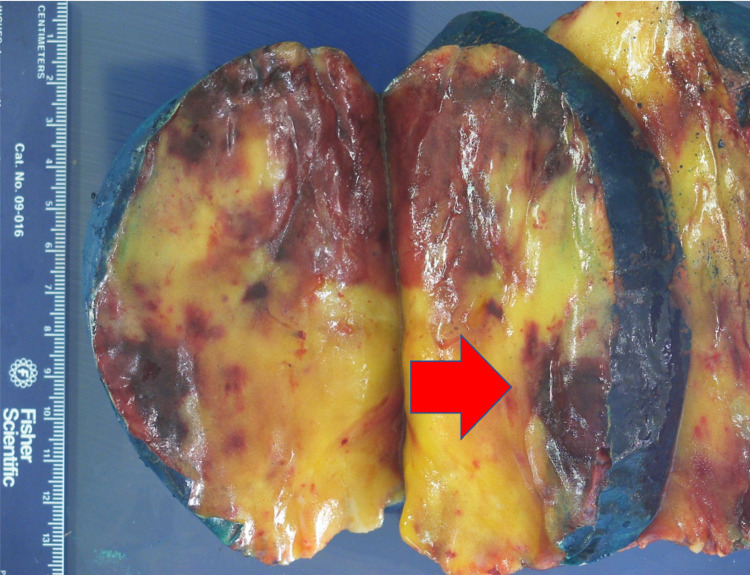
Gross Sectioned Myelolipoma Image demonstrates areas of hemorrhage (red arrow) correlating with the CT and MRI images.
